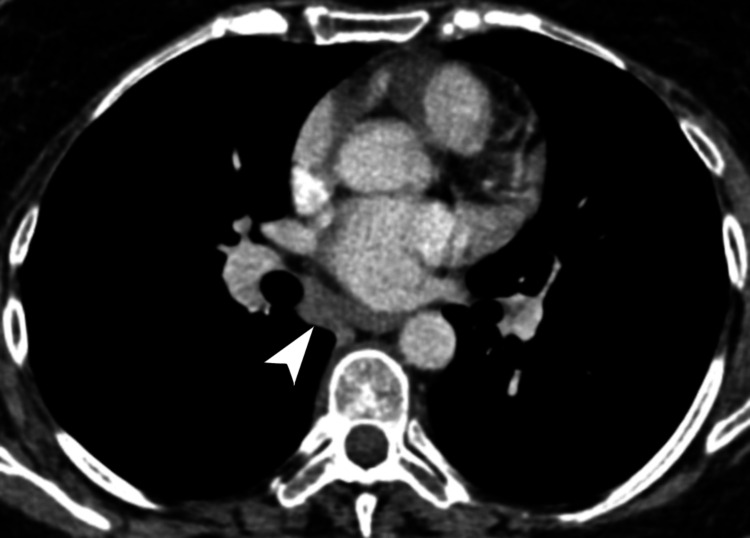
Reduction of subcarinal nodal mass at five-month follow-up. Axial CT of the chest obtained at five-month follow-up demonstrates a residual subcarinal nodal mass (white arrowhead) measuring 3.9 × 1.4 × 4.3 cm (transverse × AP × craniocaudal) with mild compression of the thoracic esophagus. CT: computed tomography
